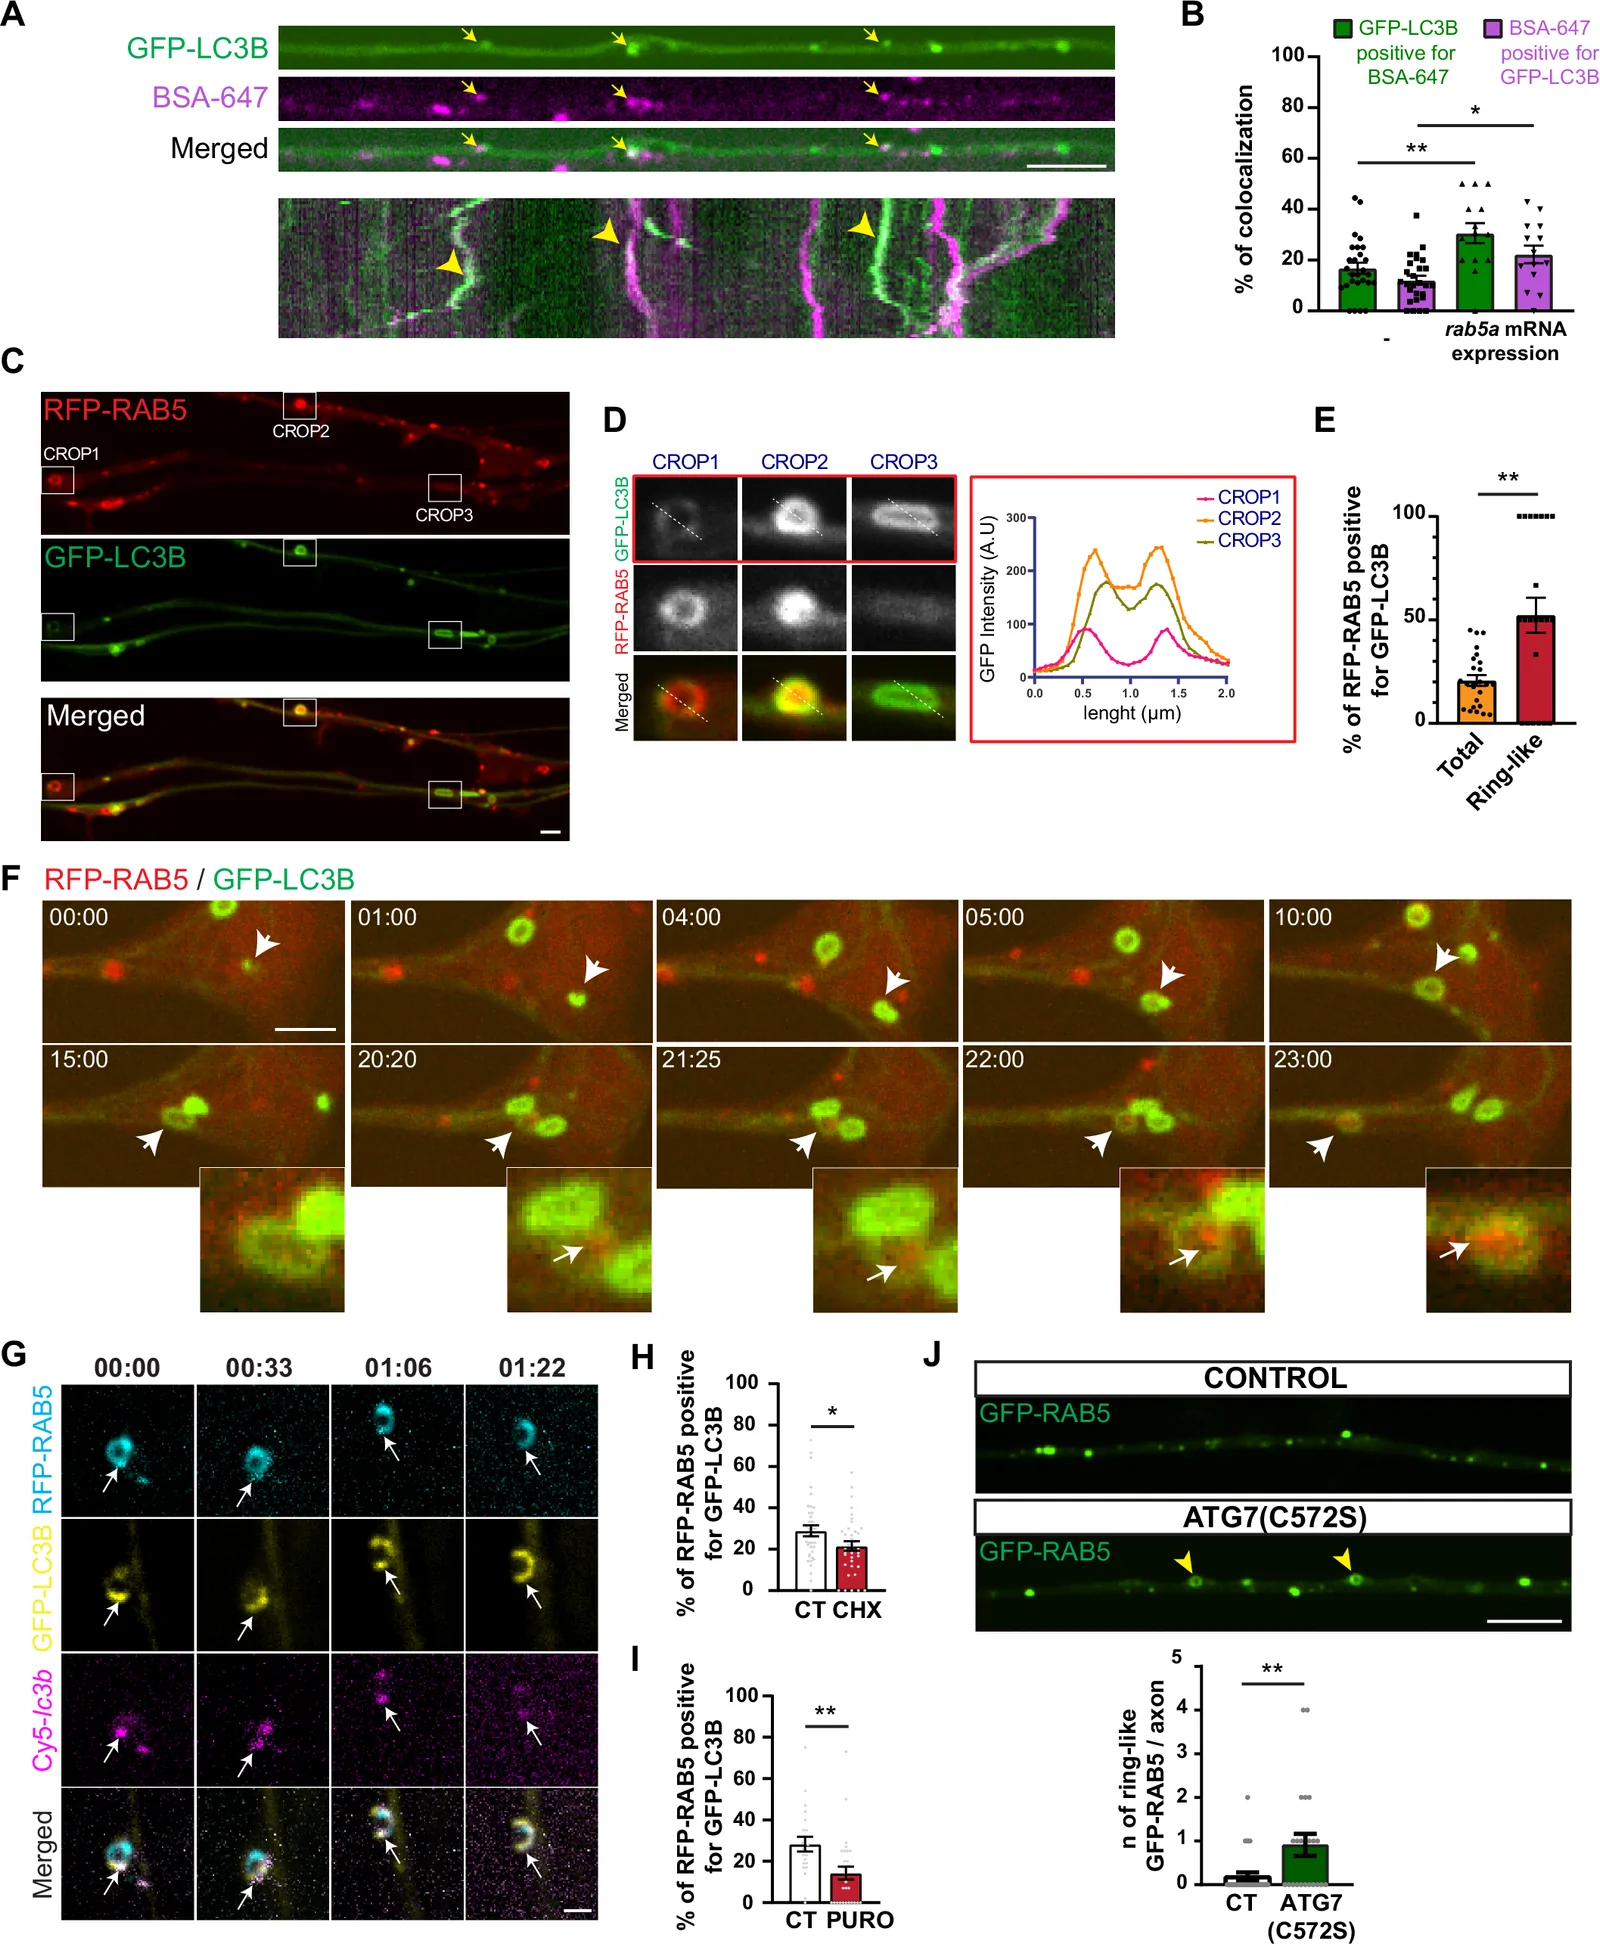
Dysfunctional endosomes generated by RAB5 overexpression become LC3B-positive, and LC3B function regulates their clearance in axons. (A) Image and kymograph (2 min) showing BSA-647 signal (in magenta) in axons expressing GFP-LC3B (in green). Arrows indicate colocalizing signals. (B) Colocalization analysis. Dots represent axons (CT: *n* = 27; RAB5 100 ng: *n* = 14, *P* = 0.0021, P* = 0.0127, *n* ≥6 explants, 3 exp). (C) Representative image showing RFP-RAB5 and GFP-LC3B signals in axons. (D) Expanded views and intensity plot of the GFP-LC3B signal along the indicated line. (E) Colocalization analysis. Dots represent axons (*n* = 25, *P* = 0.0091, *n* ≥6 explants, 3 exp). (F) Time-lapse sequence showing an axon expressing RFP-RAB5 and GFP-LC3B. Arrows indicate nascent AP in the growth cone, in which RAB5 signal accumulates. (G) Time-lapse sequence of RFP-RAB5 (in blue), GFP-LC3B (in yellow) and Cy5-*gfp-lc3b* mRNA (in magenta) signals. Arrows indicate colocalization between signals. (H) Colocalization analysis. Dots represent axons. (CT: *n* = 38, CHX: *n* = 37, *P* = 0.0485, *n* ≥4 explants, 2 exp). (I) Colocalization analysis. Dots represent axons. (CT: *n* = 22, PURO: *n* = 29, *P* = 0.0011, *n* ≥6 explants, 3 exp). (J) Images and quantification of GFP-RAB5 signal. Arrows indicate ring-like endosomes. Dots represent axons (CT: *n* = 31, ATG7(C572S): *n* = 23, *P* = 0.0038, *n* ≥6 explants, 3 exp). Mean ± SEM. (B, E, H, I, J) Mann–Whitney test. Scale bars = (A, J) 5 μm, (G) 1 μm, and (C, F) 2 μm. Time is indicated as min:sec. Source data are available online for this figure.
